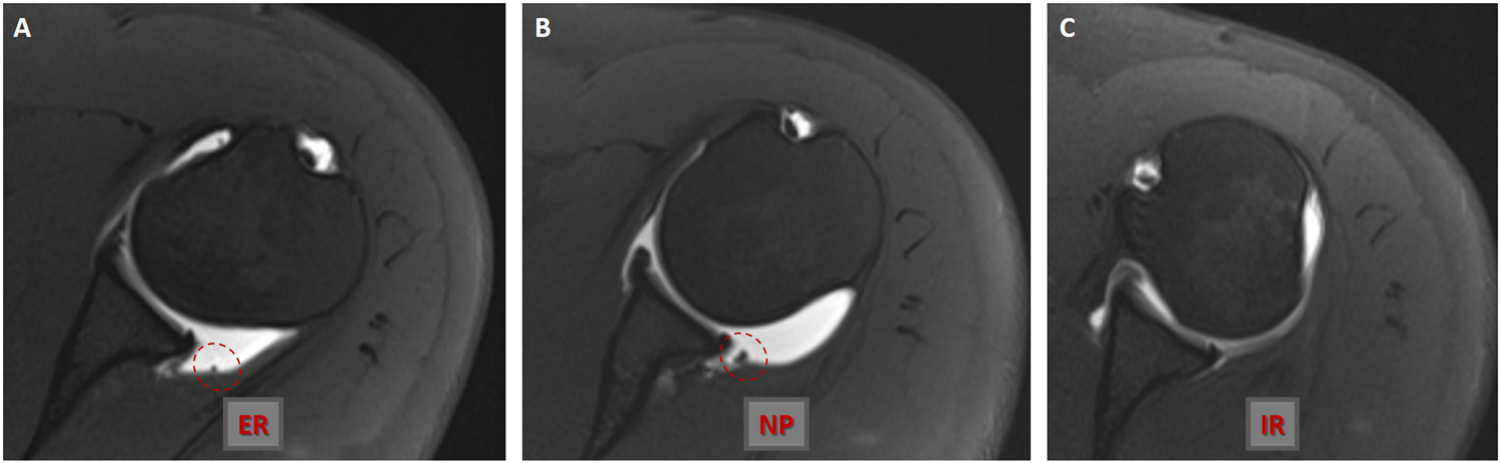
Axial fat-suppressed TSE T1-weighted MR arthrograms (A and B) obtained at the same level in the external rotation and neutral position of the ipsilateral arm show a synovial plica (circle) in the posterior shoulder joint capsule. There is no synovial fold in axial fat-suppressed TSE T1-weighted MR arthrogram (C) obtained at the same level in the internal rotation of the ipsilateral arm. ER, external rotation; IR, internal rotation; NP, neutral position
